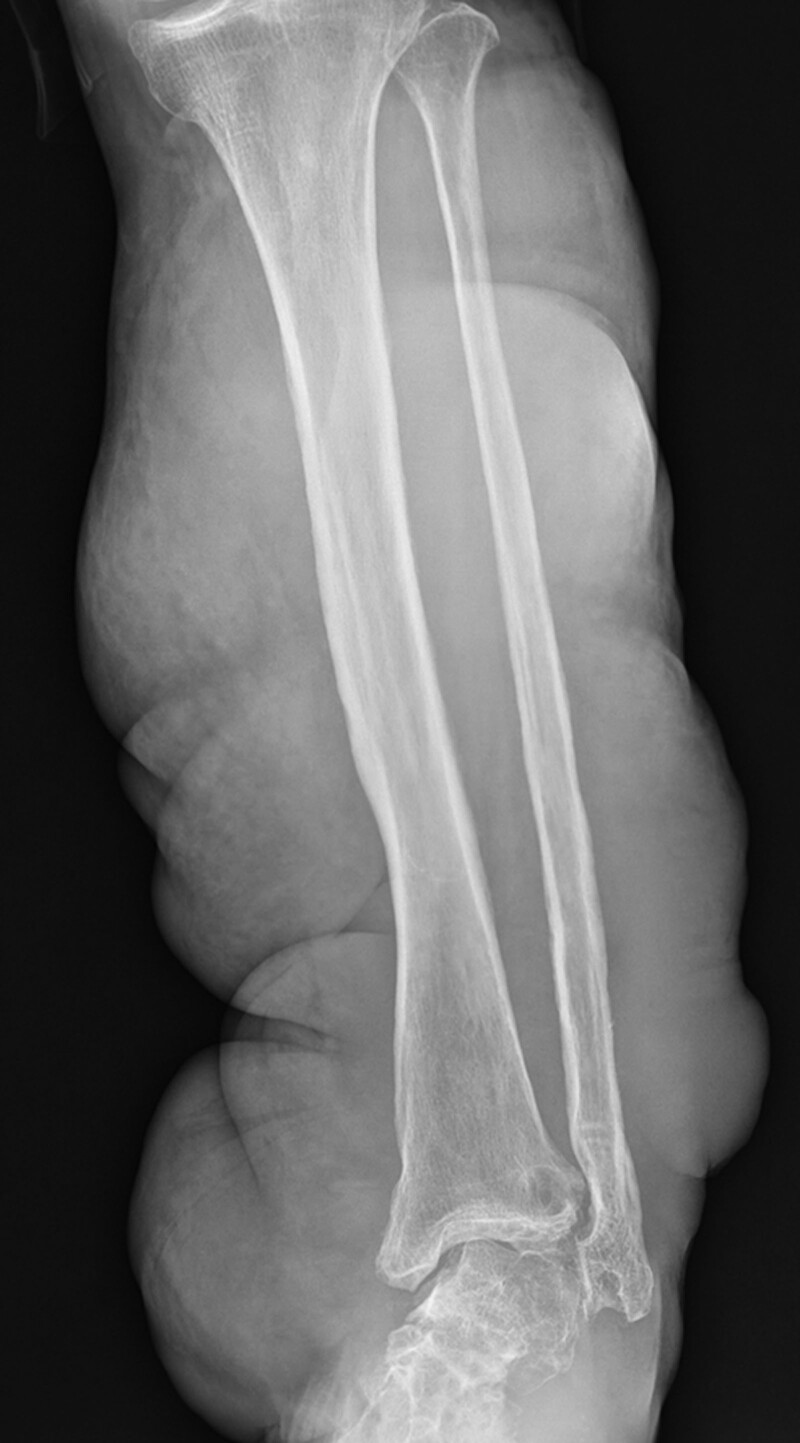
Left leg radiograph showing massive enlargement of soft tissues with cortical irregularity of the tibia and fibula.
